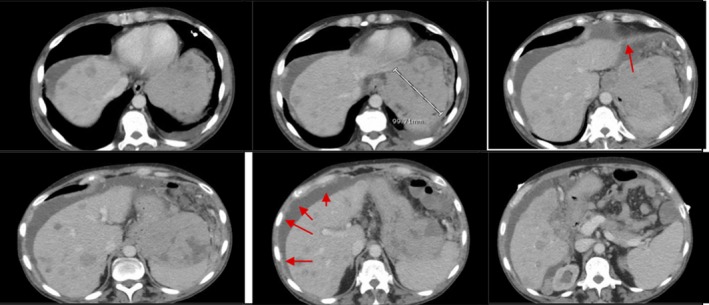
CT scan of the abdomen and pelvis with enteric contrast and intravenous contrast, 96 mL Omnipaque. Only transverse views are shown here. Approximately 7 months after images shown in Figure 1 were taken, a repeat CT abdomen and pelvis revealed new hypodense liver lesions. The above images were taken approximately 11 months after the images in Figure 1 and reviewed as an interval increase in size and number of hypodense hepatic masses. There is also evidence of ascites (arrows). In this image, the perigastric mass also appears to have enlarged.
